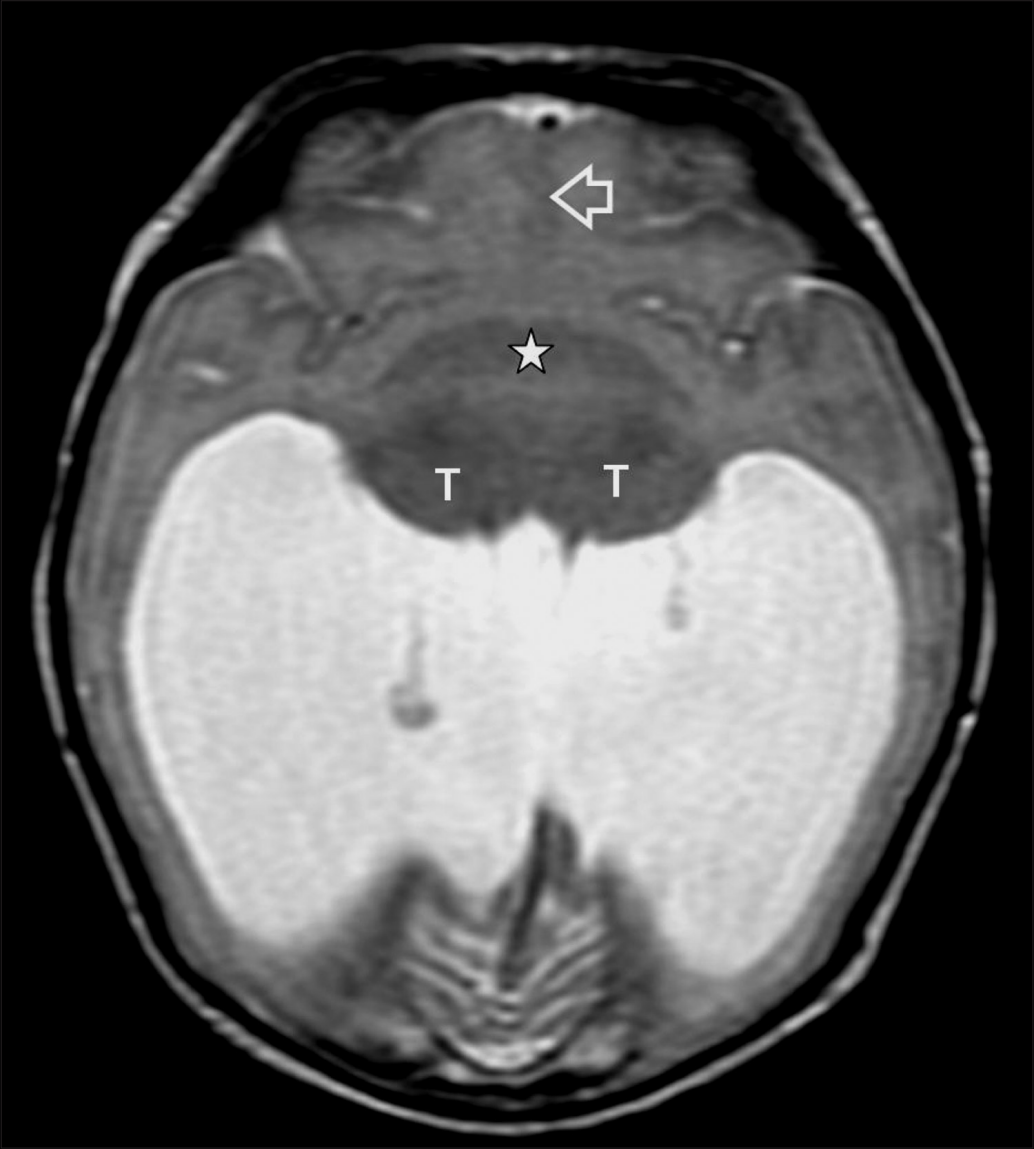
Holoprosencephaly: Axial T2W MRI of the brain at the level of the thalami shows a monoventricle and fusion of the thalami (T), basal ganglia (asterisk), and the frontal lobes, with an absent falx cerebri (open arrow)
